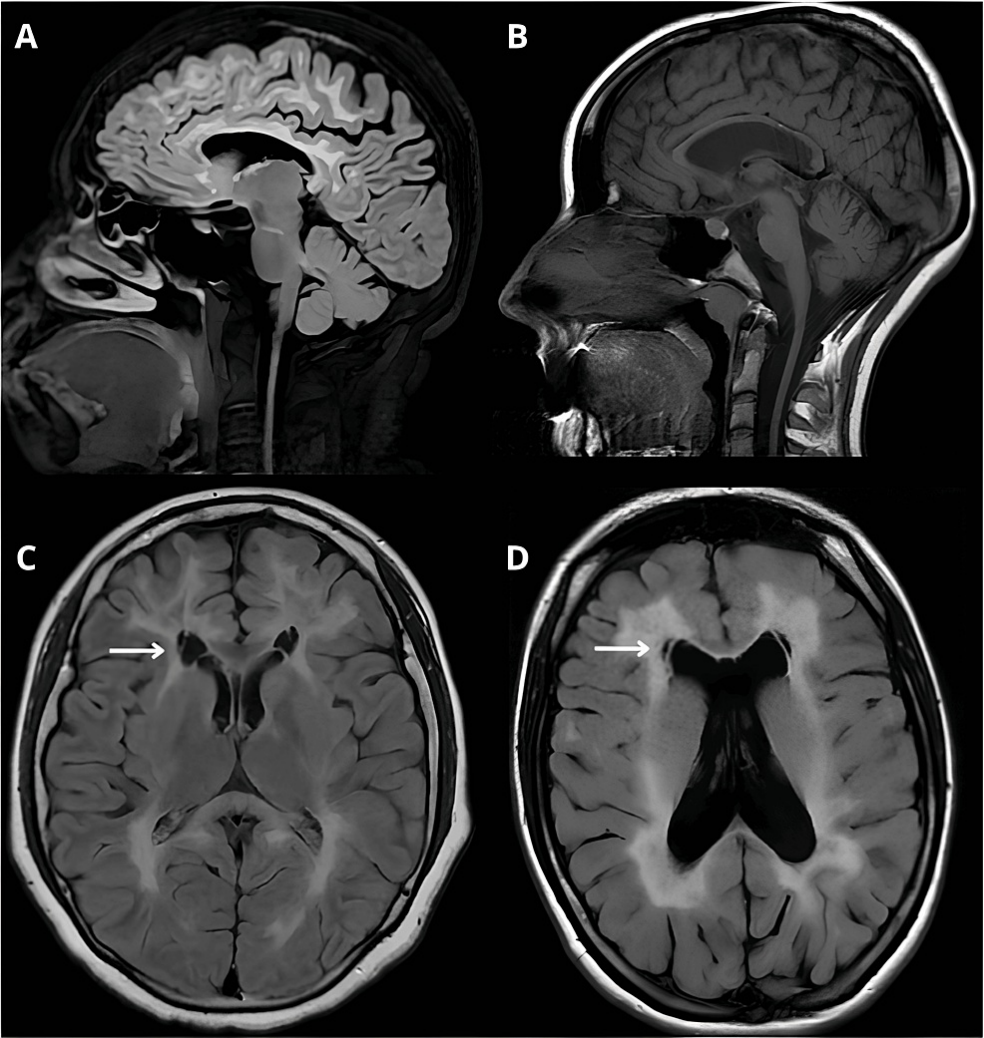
Neuroimaging findings in ovarioleukodystrophy due to alterations in the EIF2B gene complex A) Parasagittal T2 FLAIR-weighted image of the patient identified in this report showing subcortical hyperintensities in the WM, generalized cortico-subcortical atrophy, and significant atrophy of the corpus callosum, and C) axial T2 FLAIR-weighted image of the same patient showing periventricular hyperintensities and cystic degeneration of the WM at the level of the frontal horns (arrow). B) and D) similar findings with generalized and corpus callosum atrophy and cystic degeneration, as reported by Rodríguez-Palmero et al. (reproduced under a CC-BY license) [22].
